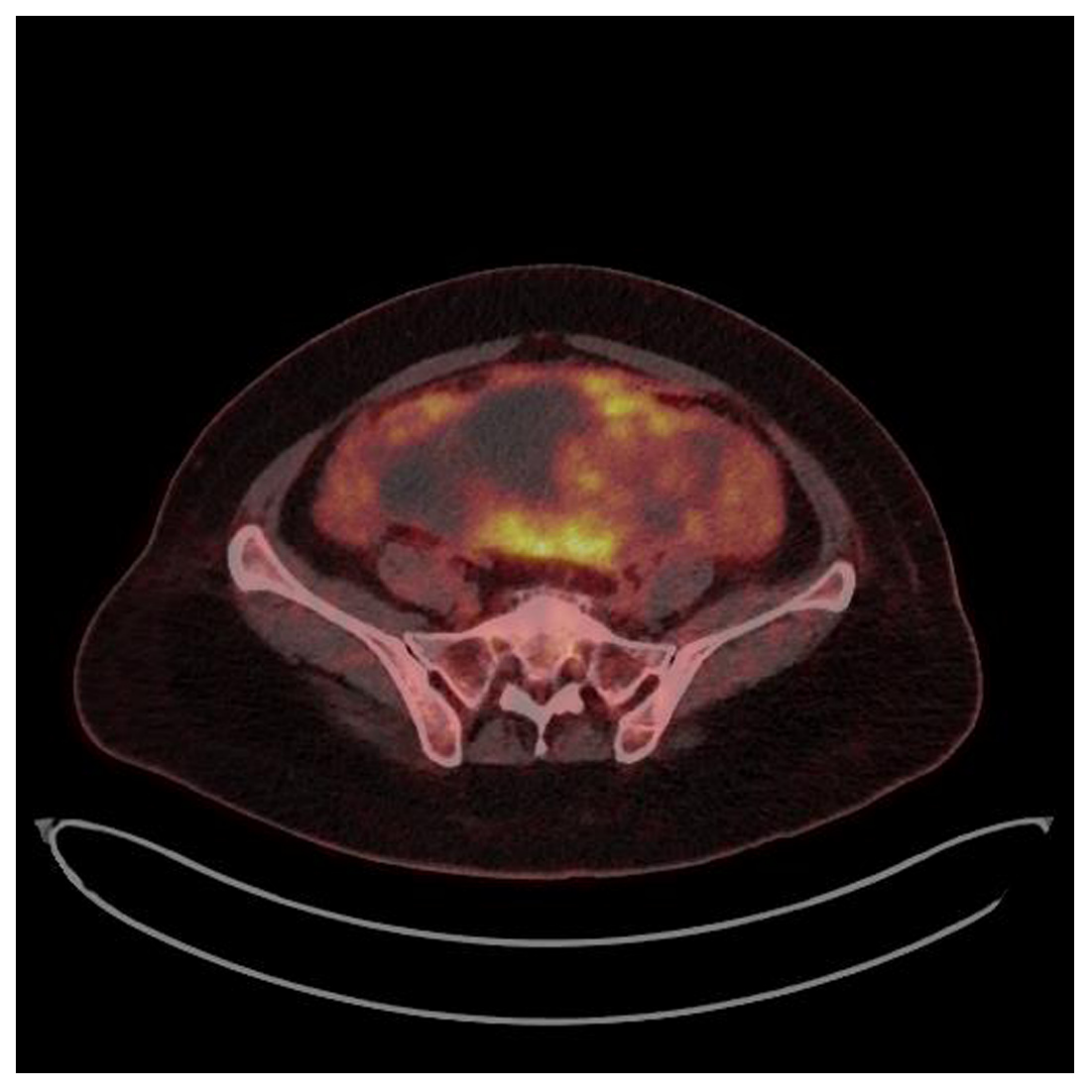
Positron emission tomography/computed tomography. An approximately 13-cm intensely hypermetabolic hemorrhagic mass is noted in the pelvic cavity, suggestive of a subserosal uterine mass possibly connected with a cervical lesion. There are regions of hypermetabolic peritoneal thickening and nodules with a small amount ascites.
